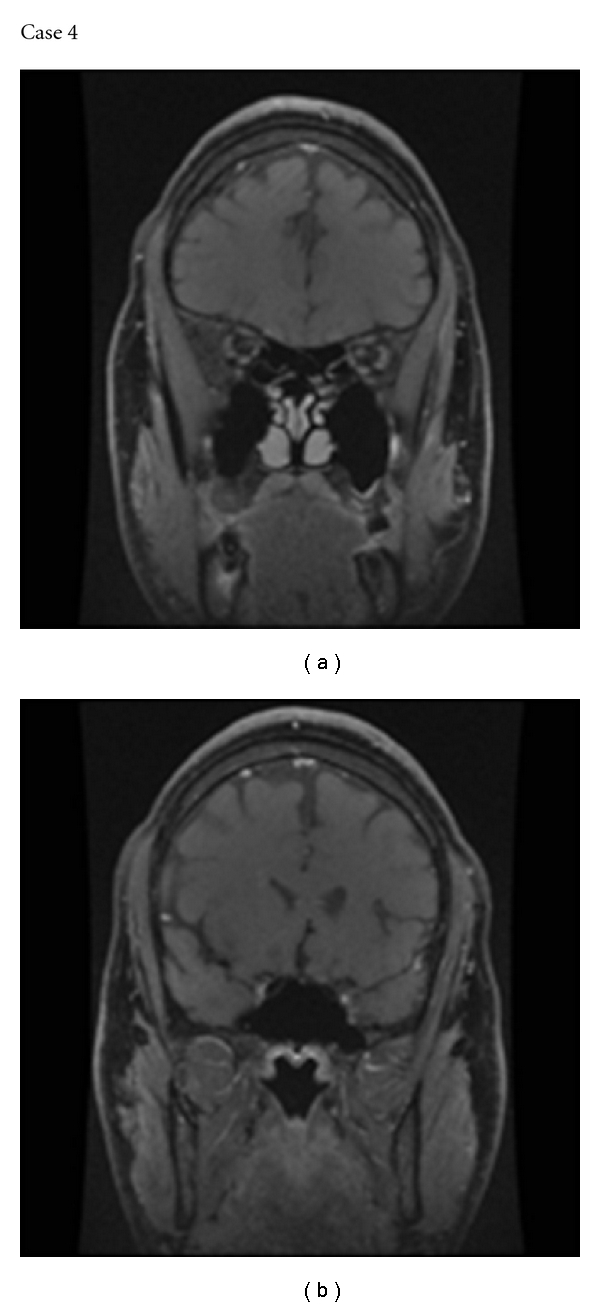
MR imaging of Case 4 showing extensive enhancement of the left optic nerve both intraorbitally (a) and intracranially (b).
